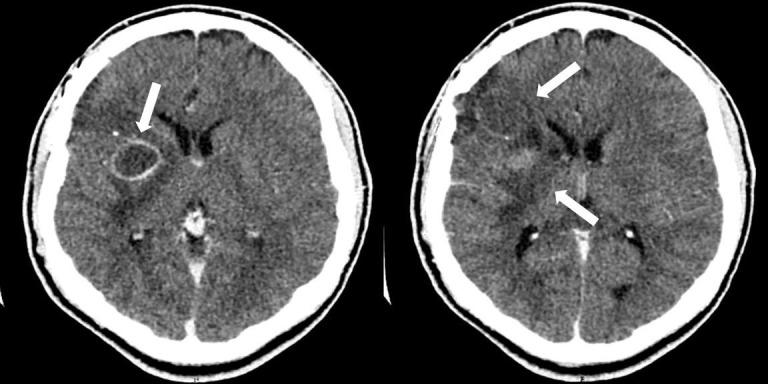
Post-operative computed tomography (CT) head with contrast performed at follow-up revealing two right frontal ring-enhancing lesions consistent with abscess.
